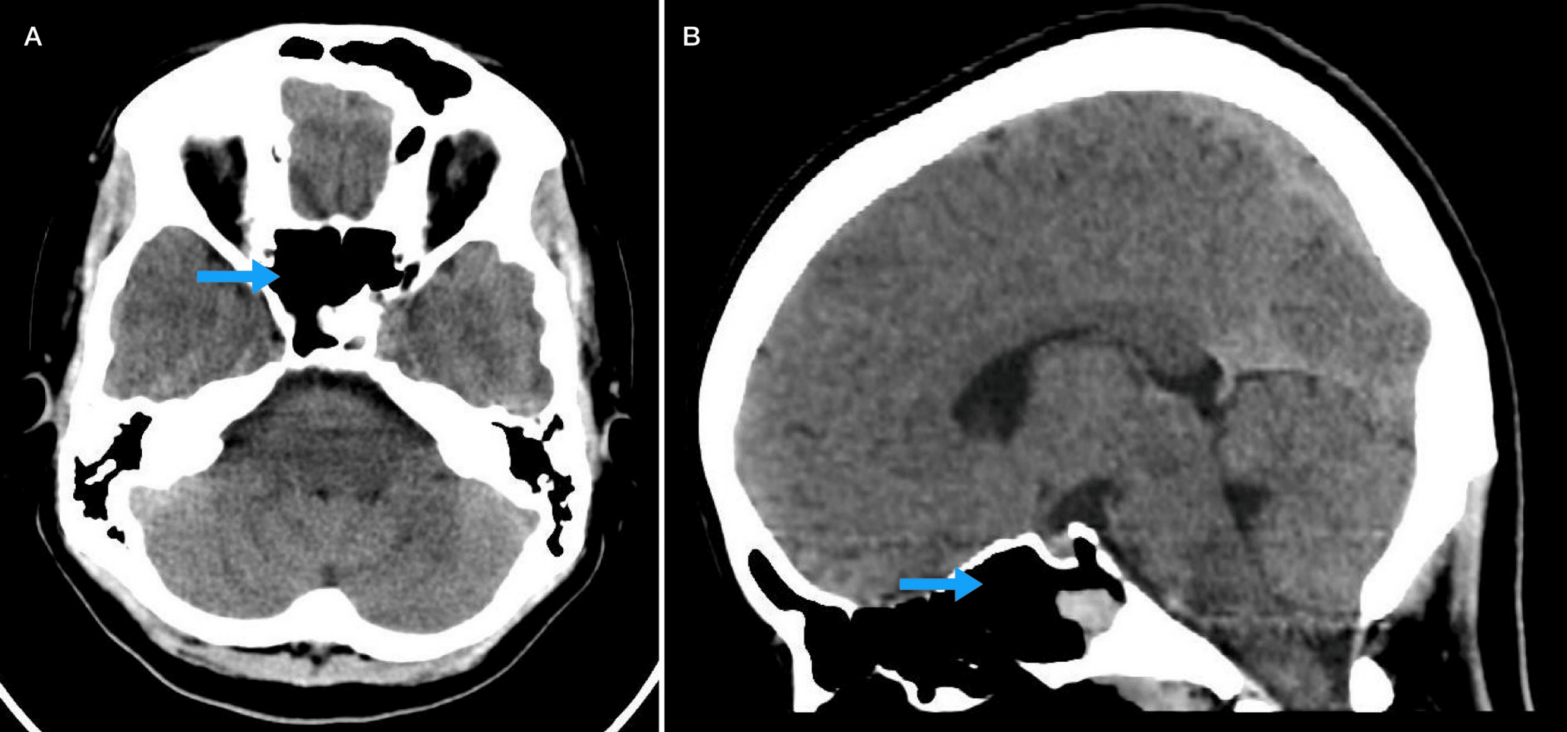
Follow-up CT brain findings. (A) Axial repeat non-contrast CT brain showing regression of the lesion (arrow). (B) Sagittal repeat non-contrast CT brain showing regression of the lesion (arrow).
